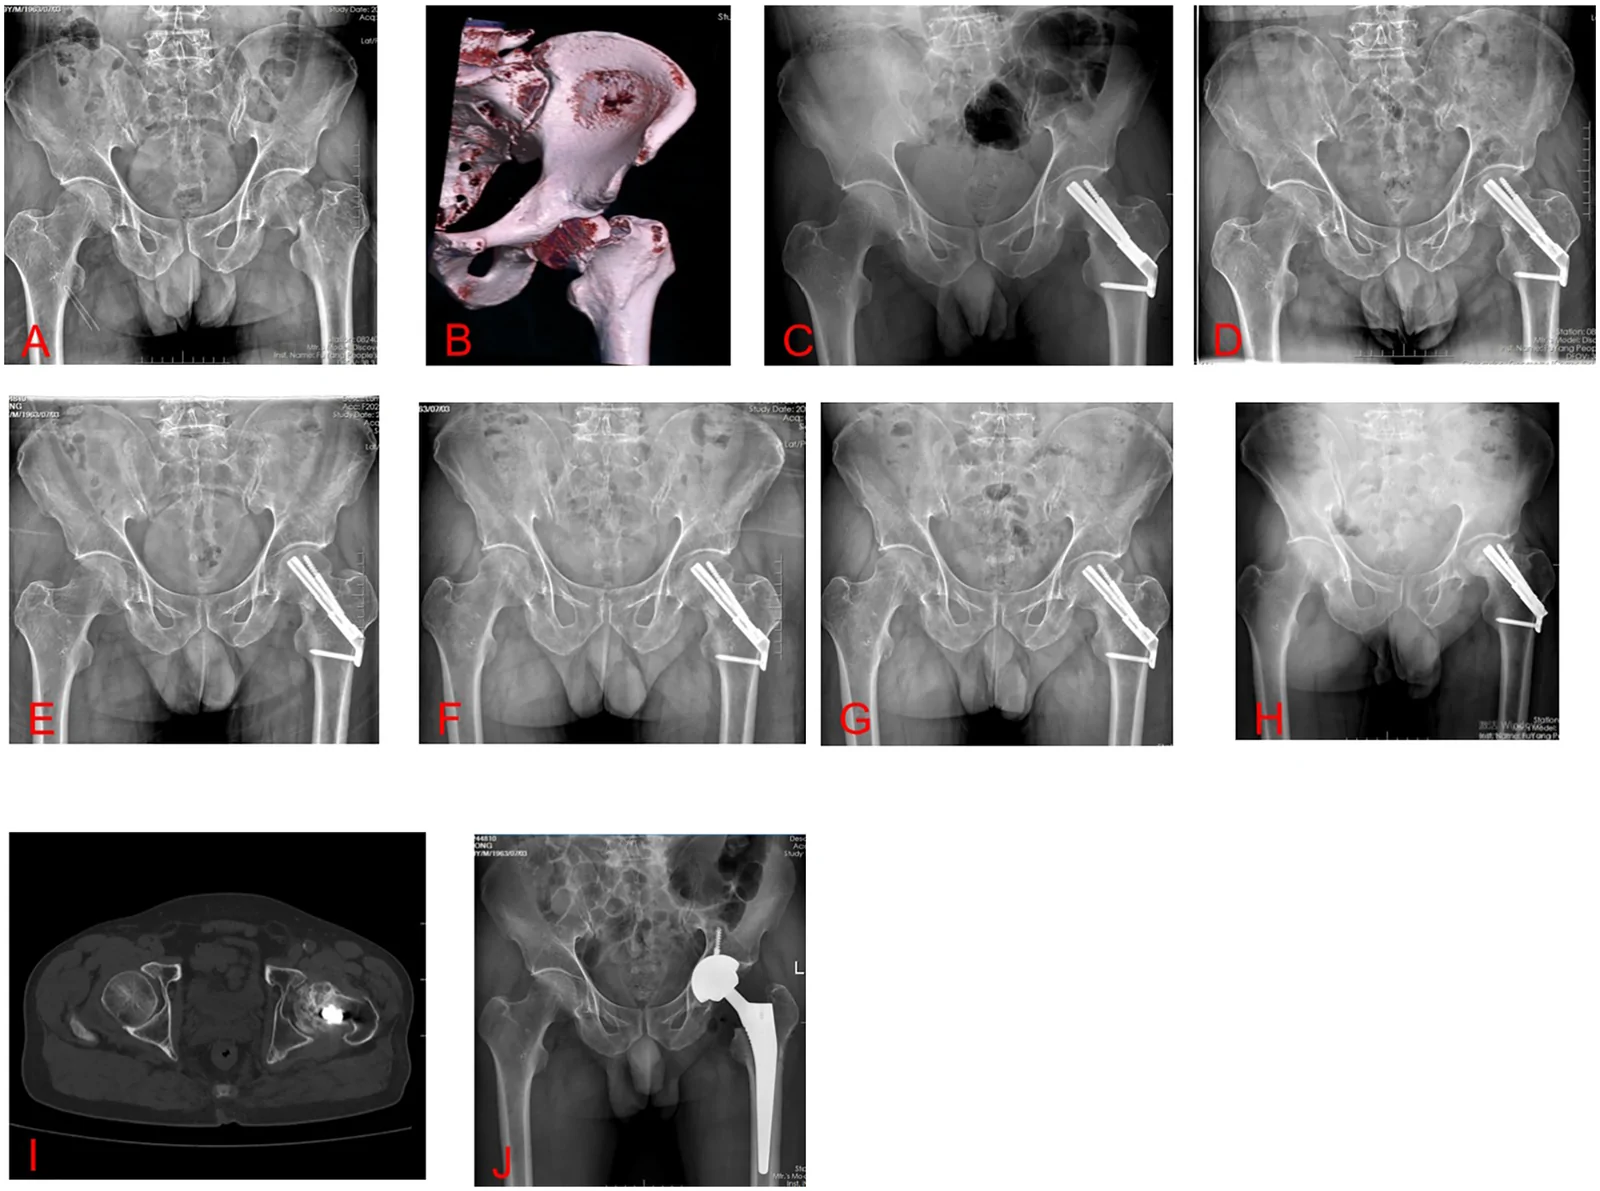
Representative case of femoral head necrosis after isolated FNS fixation. A 52-year-old male patient with a left Garden type III femoral neck fracture underwent internal fixation using the Femoral Neck System. Preoperative anteroposterior radiograph (A) and three-dimensional CT reconstruction (B) Immediate postoperative anteroposterior radiograph after FNS fixation (C) Follow-up radiographs at 1, 2, 3, and 12 months after surgery (D–G). Radiograph demonstrating femoral head necrosis at 16 months postoperatively (H), CT evaluation (I), and subsequent conversion to total hip arthroplasty (J). CT, computed tomography; FNS, Femoral Neck System; Garden classification, Garden fracture classification.
